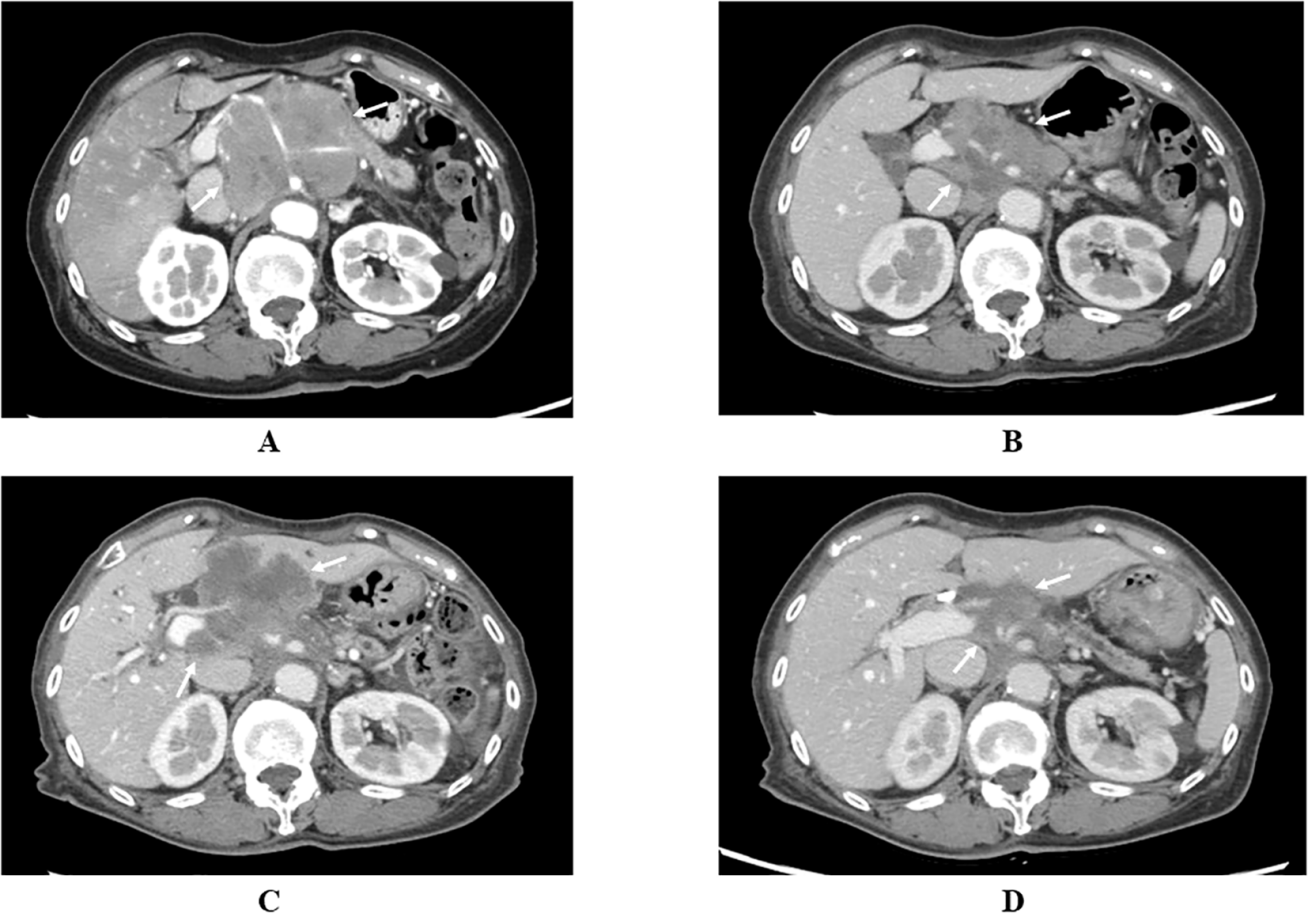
(A) Contrast-enhanced abdominal computed tomography showing a 77-mm hypovascular mass with celiac artery invasion in the pancreatic body (white arrows). (B) Significant reduction in pancreatic tumor size, indicating partial response, after two months of treatment with nab-paclitaxel plus gemcitabine (white arrows). (C) Enlargement of the pancreatic tumor with obstructive jaundice after six months of treatment (white arrows). (D) Marked reduction in pancreatic tumor size, nearly achieving partial response, four months after initiating pembrolizumab treatment (white arrows).
